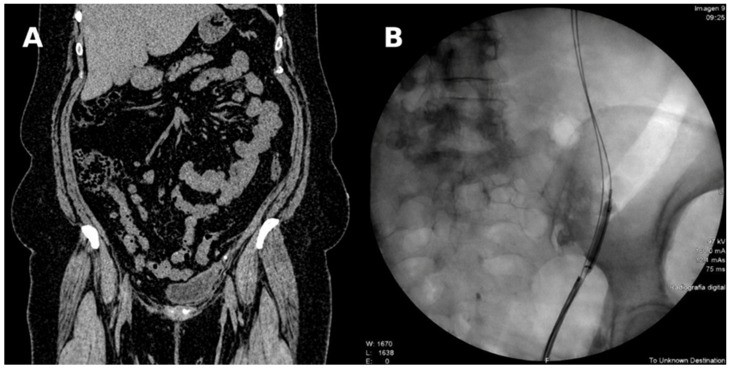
Initial evaluation and endourological treatment of the left distal ureteral stricture. (A) Non-contrast coronal abdominopelvic CT scan showing stones in the left pelvic ureter associated with grade IV ureterohydronephrosis and severe dilatation of the collecting system. (B) Intraoperative fluoroscopic image during ureteroscopy with pneumatic dilation and endoscopic incision of the distal ureteral stricture, with placement of a double-J stent.
